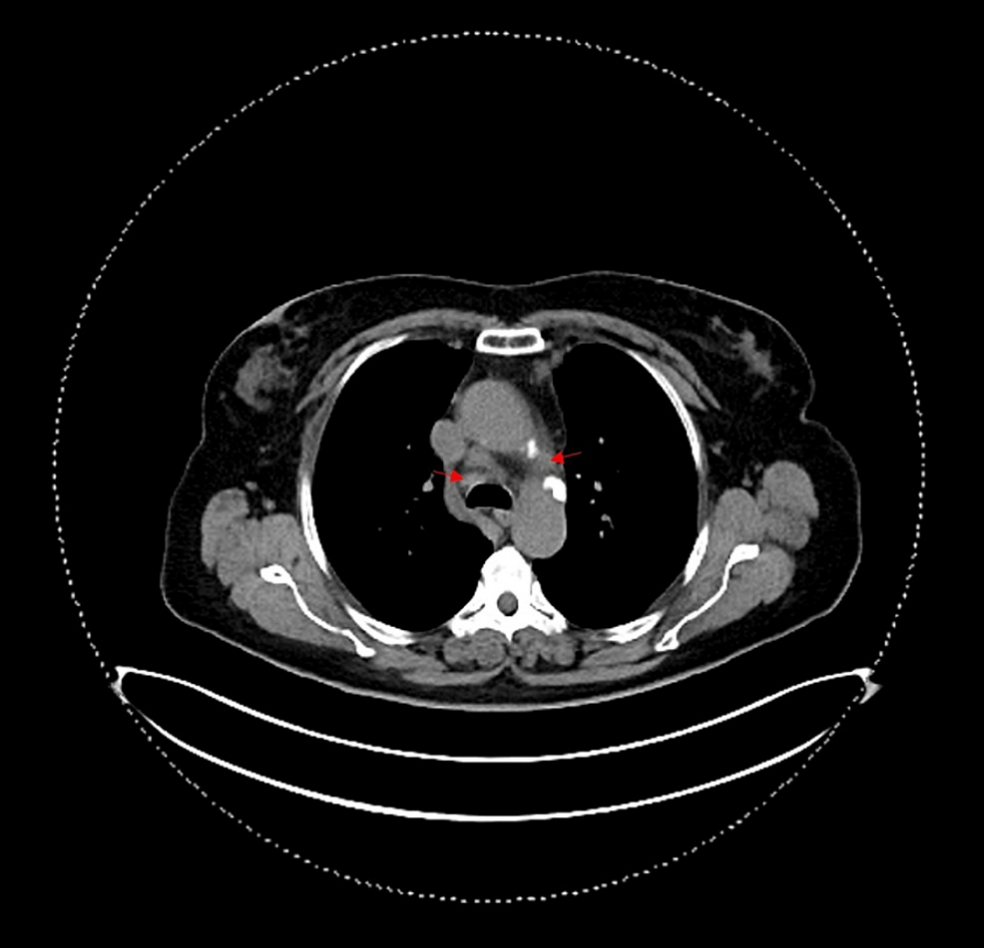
Thoracic CT Red arrows - mediastinal lymphadenopathies
